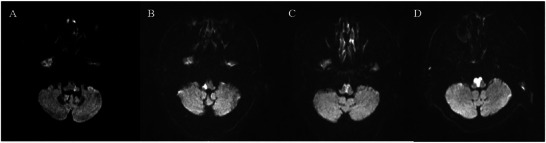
Specific lesion patterns in medullary infarction with respiratory failure on DWI. (A) Typical in LMI, (B) large in LMI, (C) Y‐shaped in MMI, and (D) heart‐shaped in MMI.
